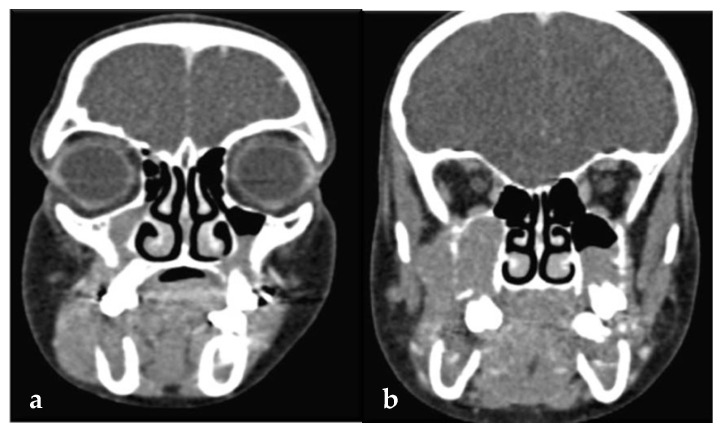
(a,b): Computed tomography showing hypodense (soft tissue) masses along and distal to the mandibular ramus extending into the maxillary sinus, bilaterally.
